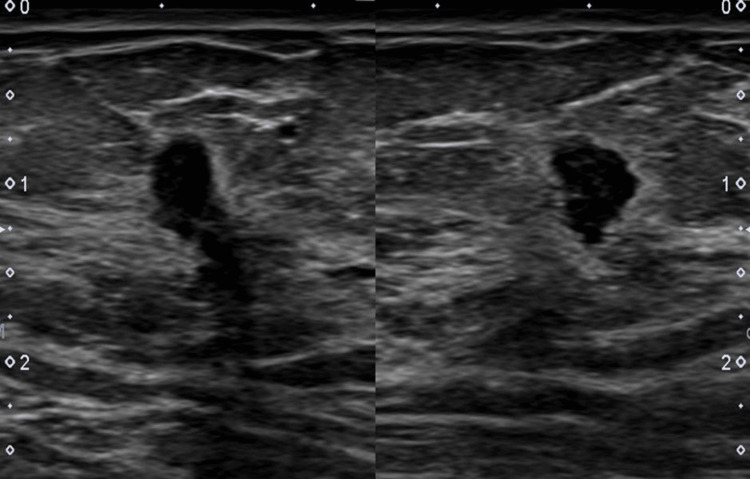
Ultrasound of the right breast showing an irregular hypoechoic mass at the 9-10 o'clock axis, 6 cm from the nipple, which measures 1.1×0.5×0.6 cm and at the 9 o'clock axis, 6 cm from the nipple, which measures 0.7×0.5×0.2 cm
